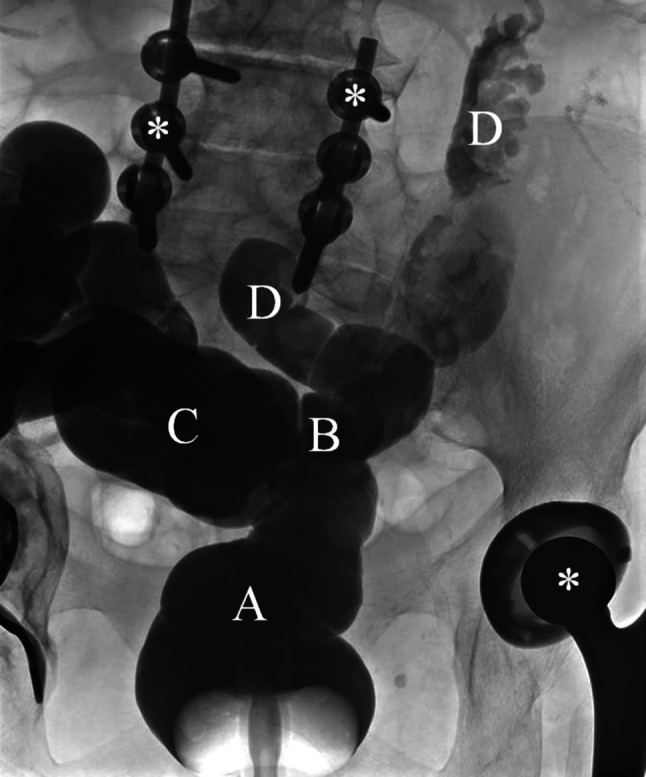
Barium enema performed with special attention in search of an abnormal connection between the colon and small intestine: *A* rectum, *B* fistula connecting the proximal rectum and small bowel, *C* descending colon, *D* small bowel, *asterisks*: metal from hip replacement and lumbosacral stabilization which caused artifacts on CT scan
